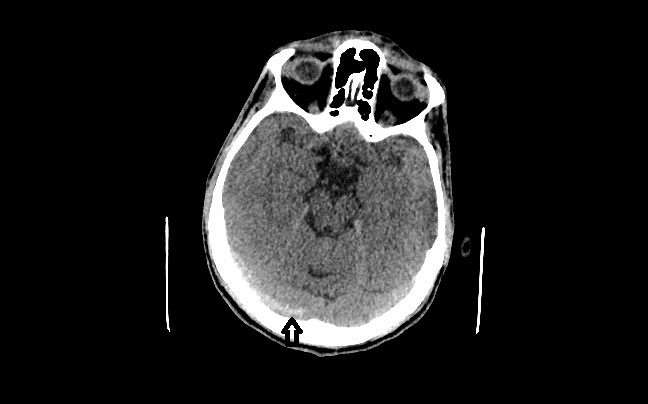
Computed tomography of the brain demonstrating hyperdensity in the region of right transverse sinus (arrow).
